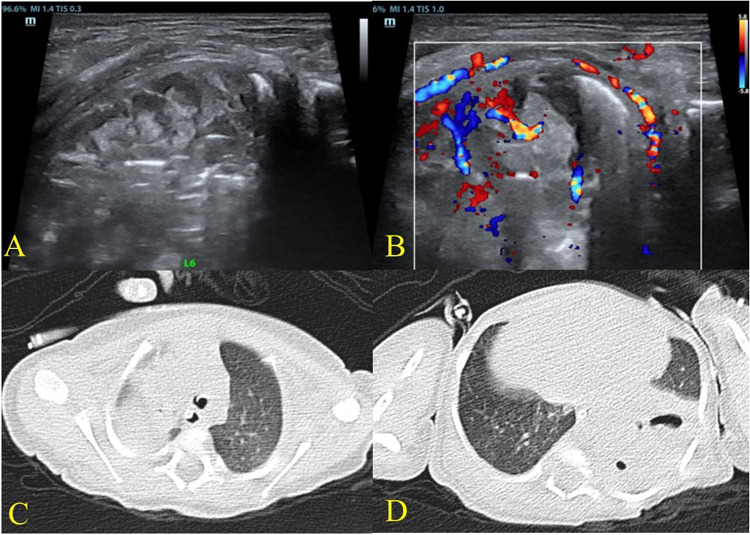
Lung ultrasound and chest CT findings on hospital day 4 demonstrating persistent left lung consolidation with suspected abscess formation. On hospital day 4, under conventional mechanical ventilation, ultrasound revealed persistent left lung consolidation with heterogeneous echotexture (A) Multiple strip-like anechoic areas without blood flow were observed within the consolidation, while vascular signals remained detectable in surrounding consolidated tissue (B), suggestive of pneumonia with partial abscess formation. Chest CT demonstrated multiple areas of ground-glass opacity and consolidation in both lungs, more prominent in the right upper lobe (C) and left lower lobe (D) Air–fluid levels were visible within the left lower lobe consolidation, consistent with inflammation and suspected pulmonary abscess formation.
